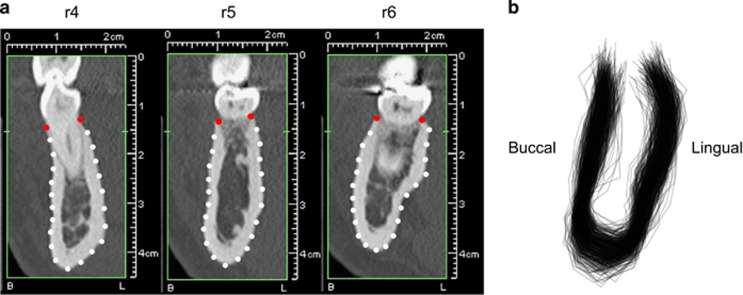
Landmark scheme for the mandibular cross-sections. (a) Cross-sectional computed tomography (CT) reconstructions at the three regions of interest: first premolar (r4), second premolar (r5) and first molar (r6) with fixed landmarks (red) and semilandmarks (white). (b) Reconstructed mandibular outlines of all 450 sections after standardizing the location and orientation of the landmark configurations.
